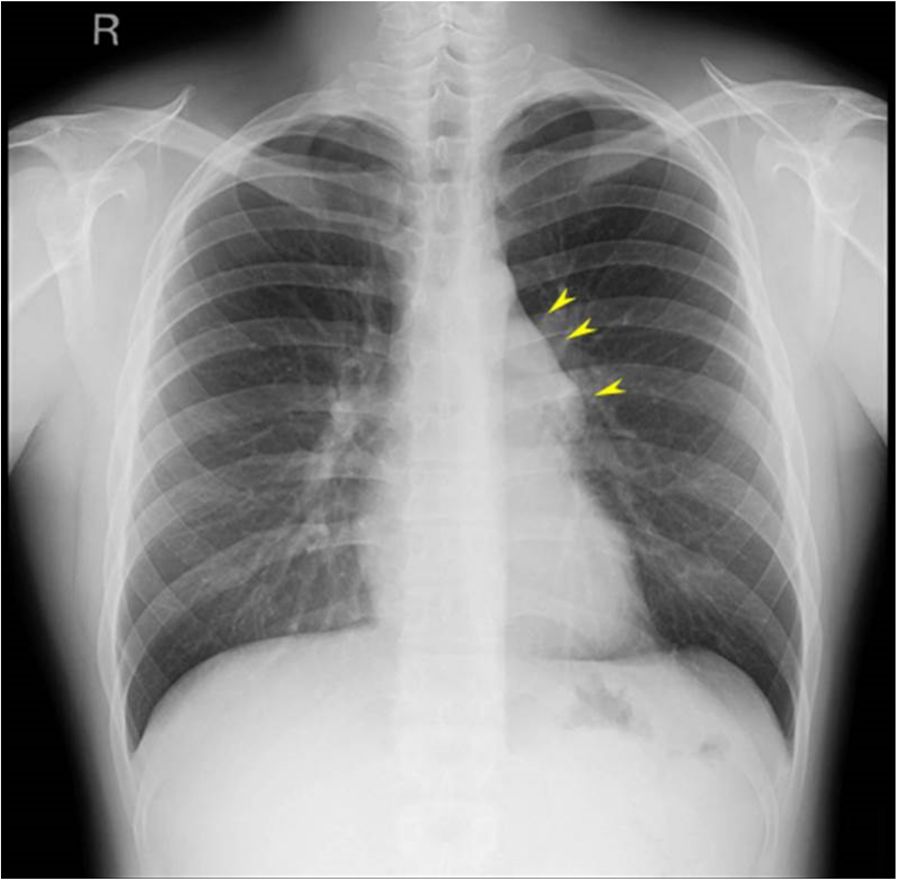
Chest X-ray showed a protrusion of the aortopulmonary window (*arrowheads*)
